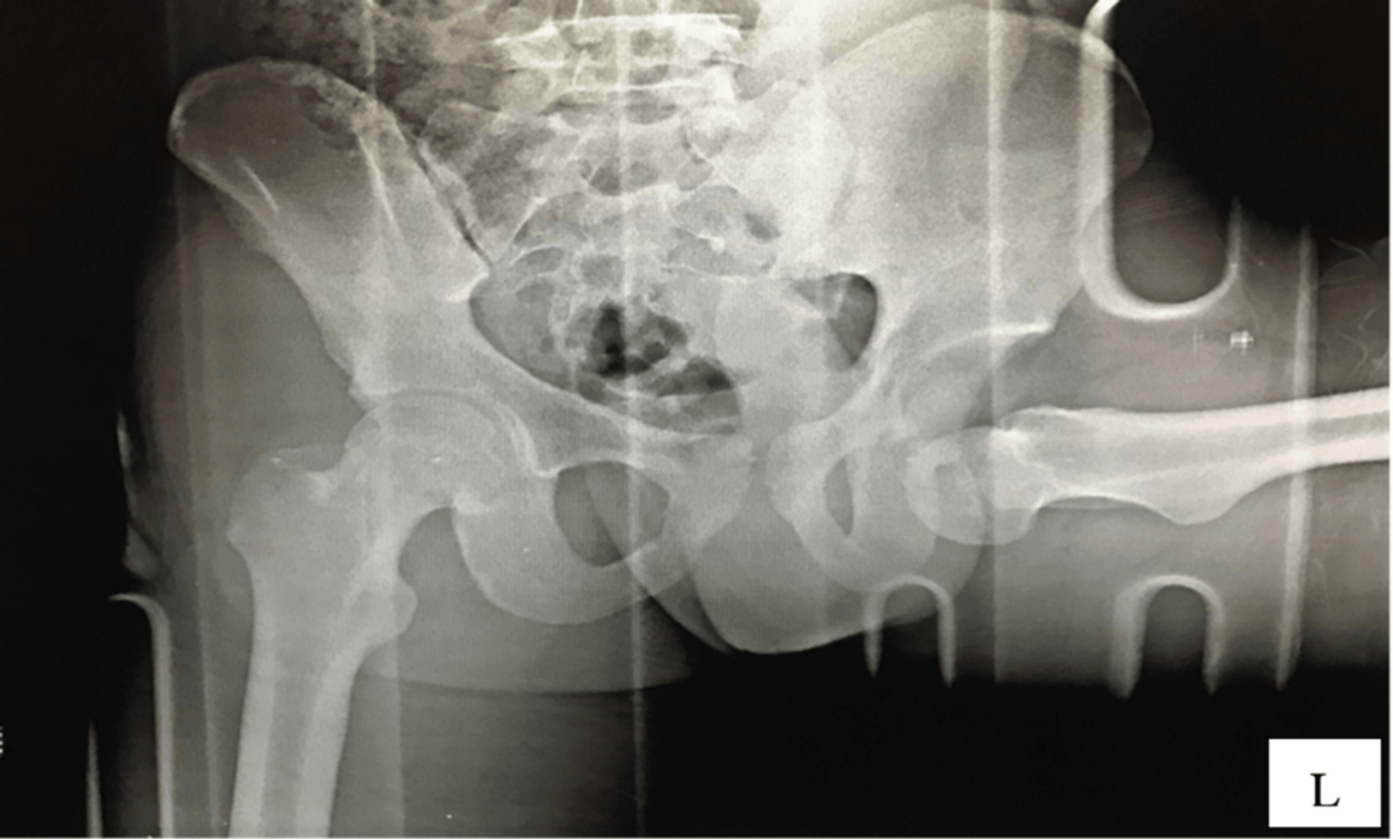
Preoperative anteroposterior radiography of the pelvis with both hips reveals an inferior-anterior dislocation of the left femur.
